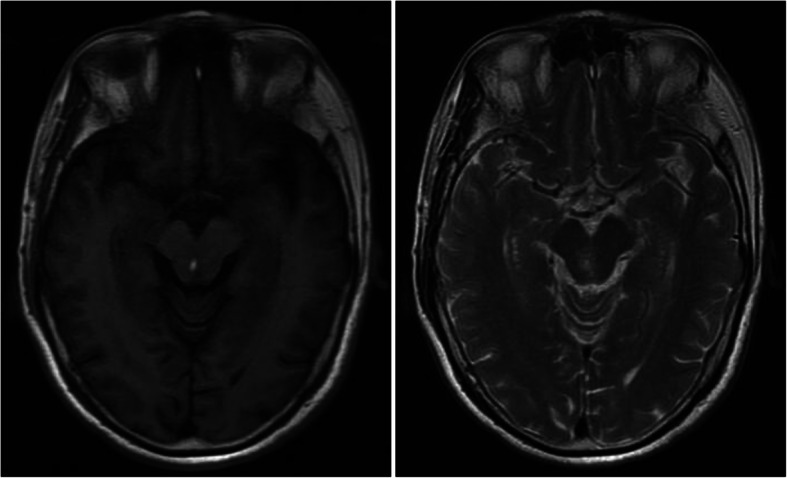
An abnormal patch signal is shown in the midbrain on cranial magnetic resonance imaging, high signal intensity on the T2-weighted imaging, and higher signal on the T1-weighted imaging
